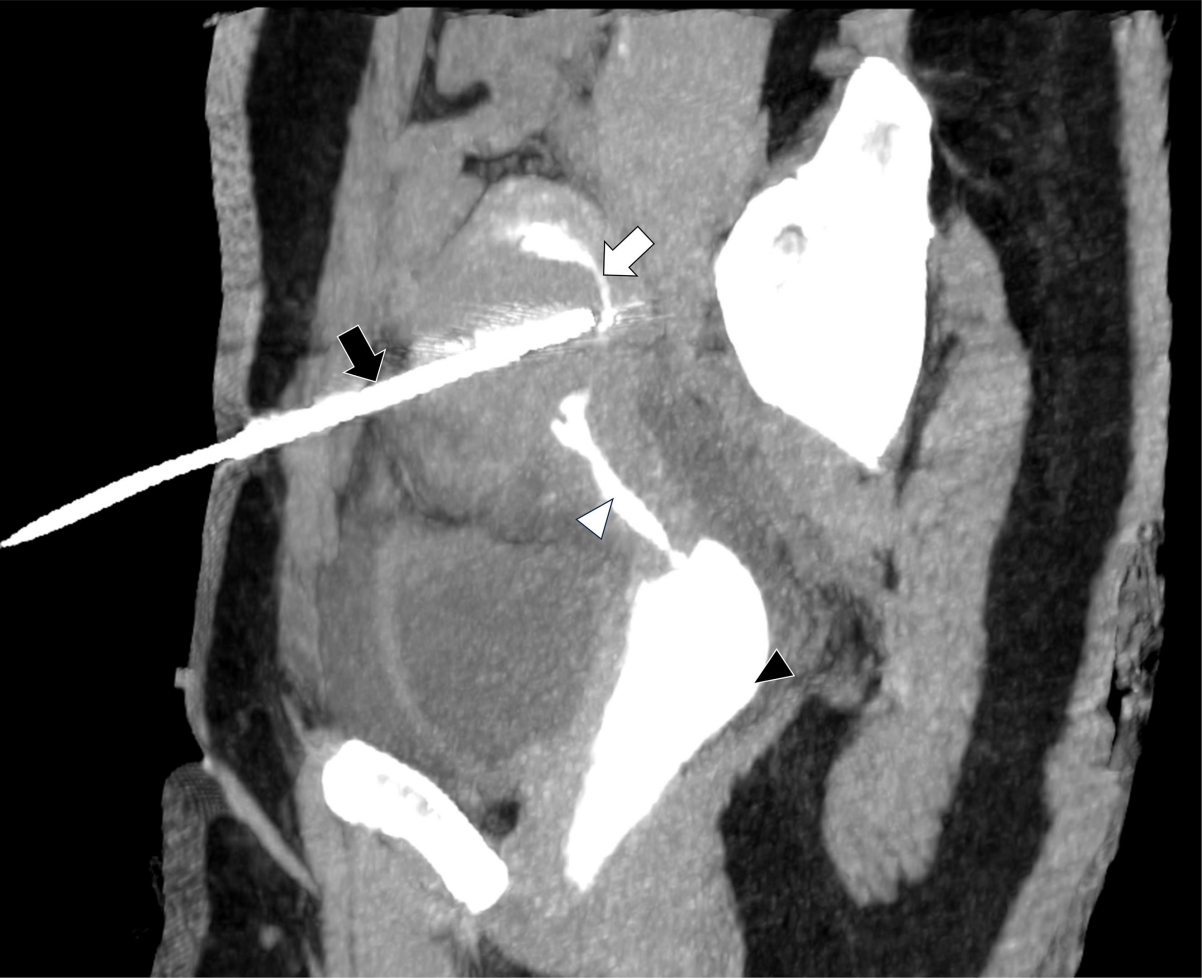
The sagittal and maximum intensity projection contrast-enhanced CT image of uterine cavity after fibroid ablation for a 50-year-old woman. The uterus cavity (white arrow), cervix (white triangle) and vagina (black triangle) is filled with normal saline containing contrast agent. The ablation antenna (black arrow) did not reach the uterus cavity. No contrast agent was observed in the pelvic cavity.
